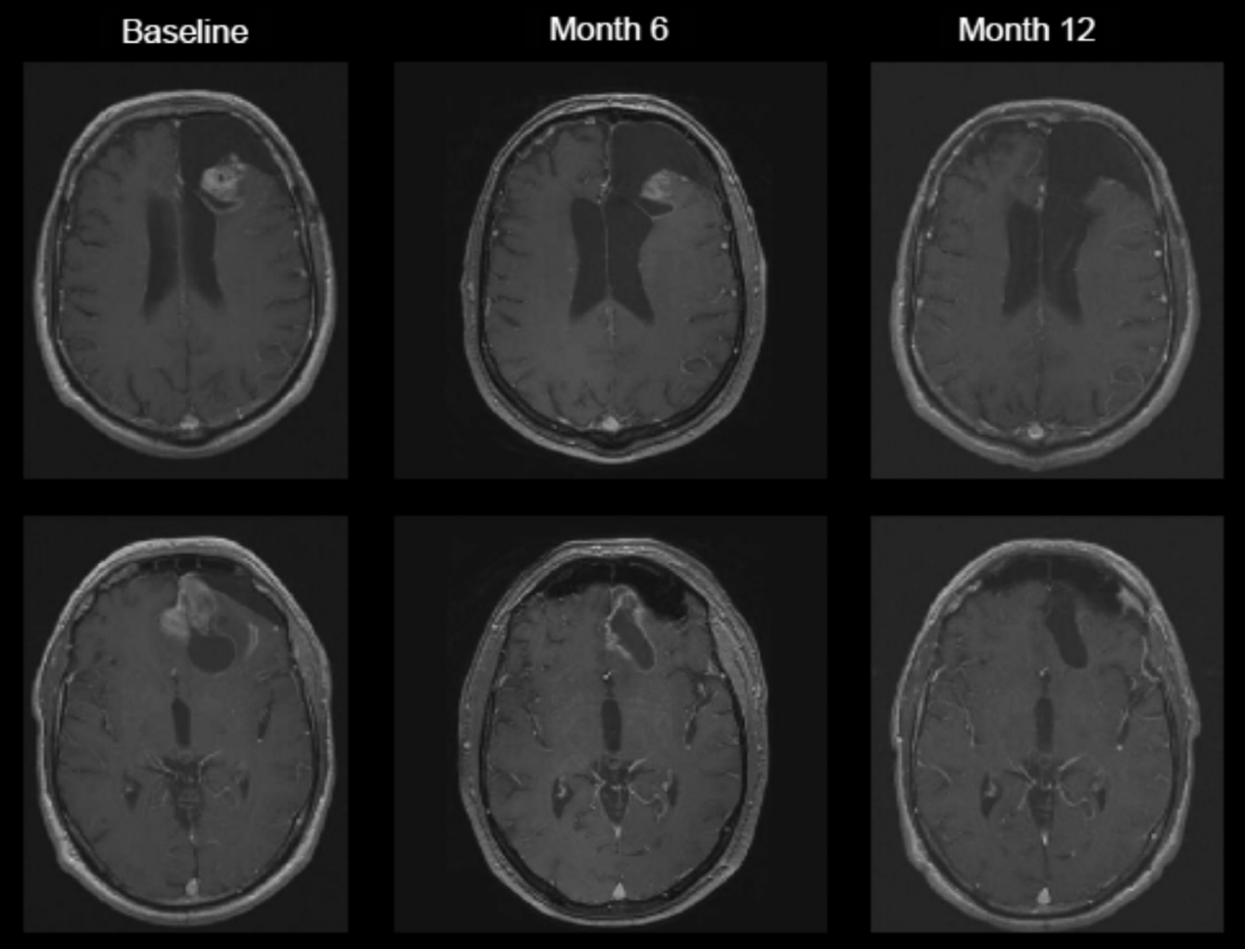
MRI from a complete responder treated with NovoTTF-100A monotherapy. Partial response was noted only after 6 months and complete response was noted after 12 months. MRI, magnetic resonance imaging.
